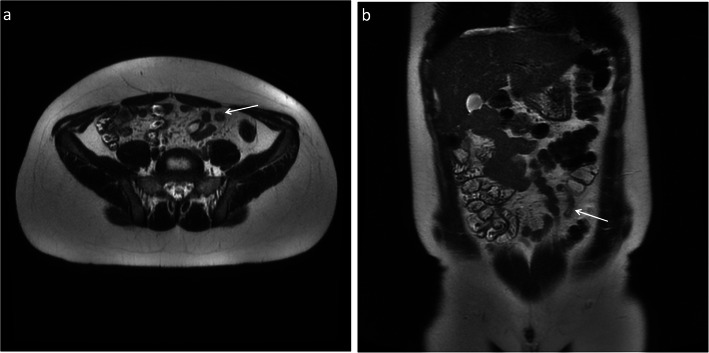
Midgut malrotation; T2-weighted MRI without fat saturation in a 12-year-old male patient demonstrating the appendix situated to the left of midline, deep to the rectus abdominis muscle. It is thickened near the tip measuring 9 mm with surrounding inflammatory fat stranding (arrows). Axial (a) and coronal (b) T2-weighted MRI images are presented, respectively
